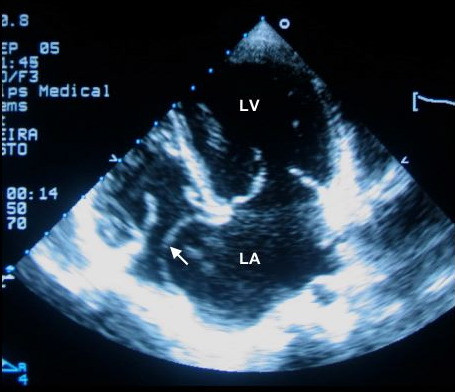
Trans-thoracic echocardiography (2-D apical, four cavities view) showing a giant left atrium (73 cm^2^). Note the marked deviation of the atrial septum (white arrow). LA: left atrium, LV: left ventricle.
